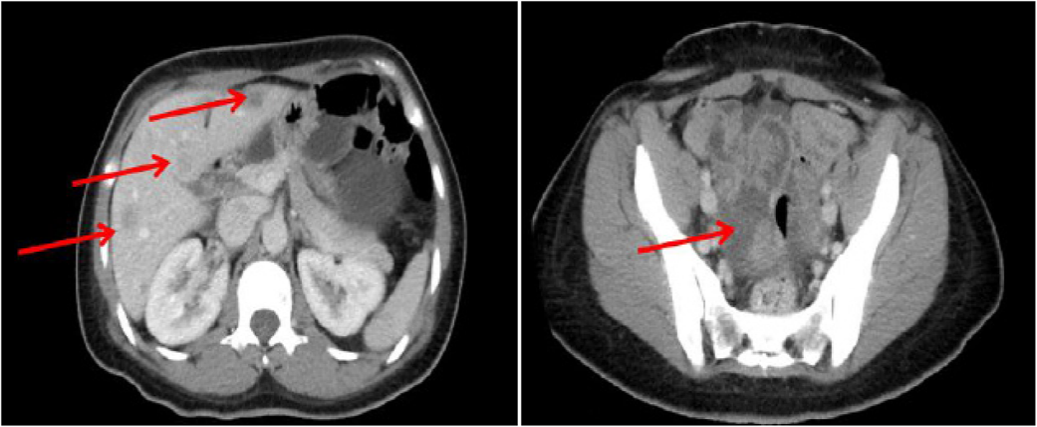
Initial staging computed tomography scan at our institution demonstrating multiple hepatic metastasis and peritoneal carcinomatosis indicated by arrows.
